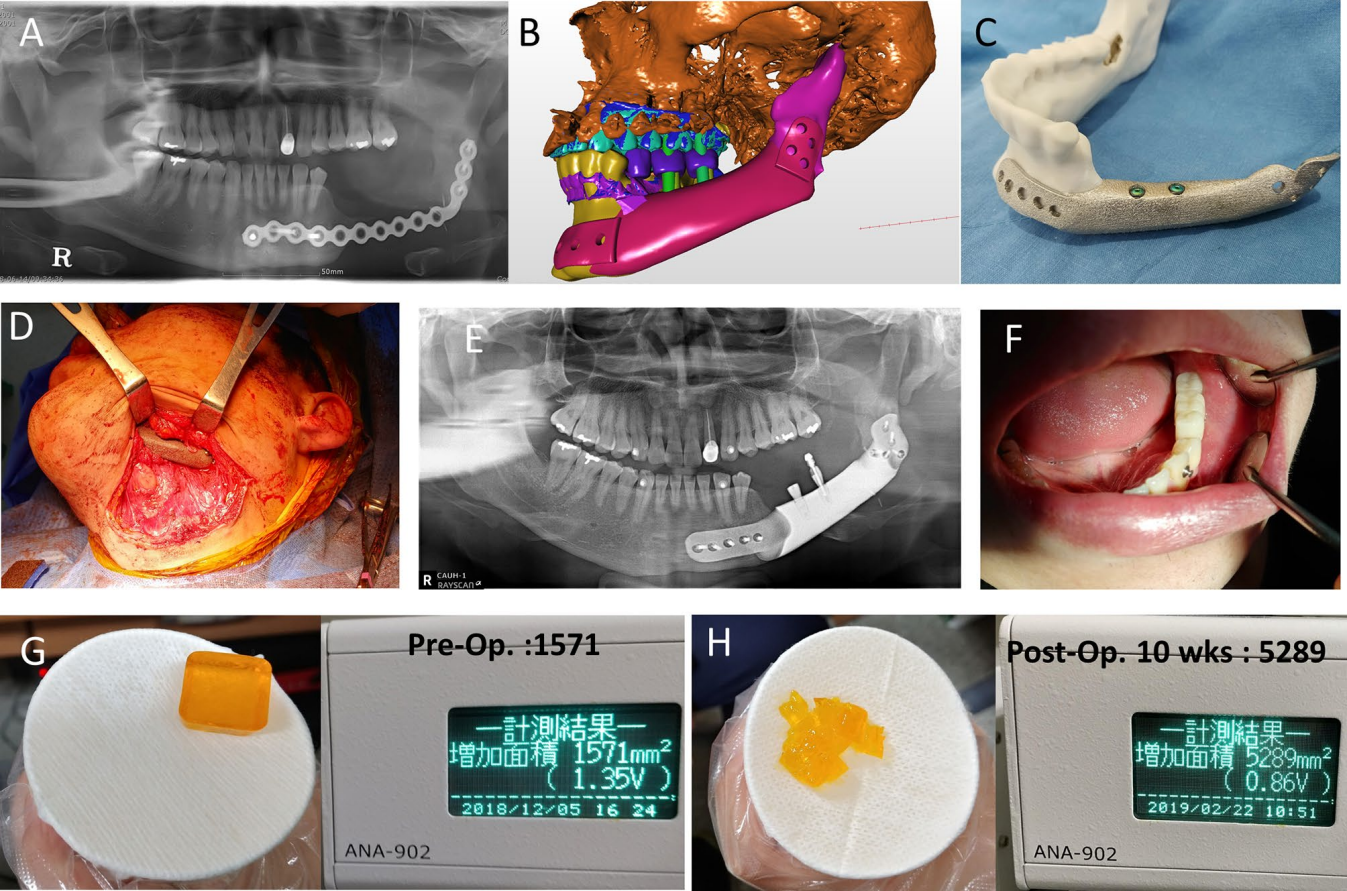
Dental implant on a titanium mandible. (A) Initial panoramic radiography of the patient (27/M); s/p ameloblastoma resection and fibula free flap failed. (B) To restore the patient’s masticatory function, two holes were designed to place dental implants. (C) Two Osstem implants were placed in the titanium mandible; (D) A titanium three-dimensional printed mandibular implant with a prefabricated dental implant was placed under general anesthesia. (E) The second implant surgery was performed 6 weeks postoperatively. (F) Prosthetic treatment was performed using a custom abutment and temporary crowns. (G, H) The yellow jelly was chewed 30 times by the patient, and the surface area was measured to see how well the jelly was chewed. The mastication efficiency measured after surgery increased three-fold compared with that before surgery.
